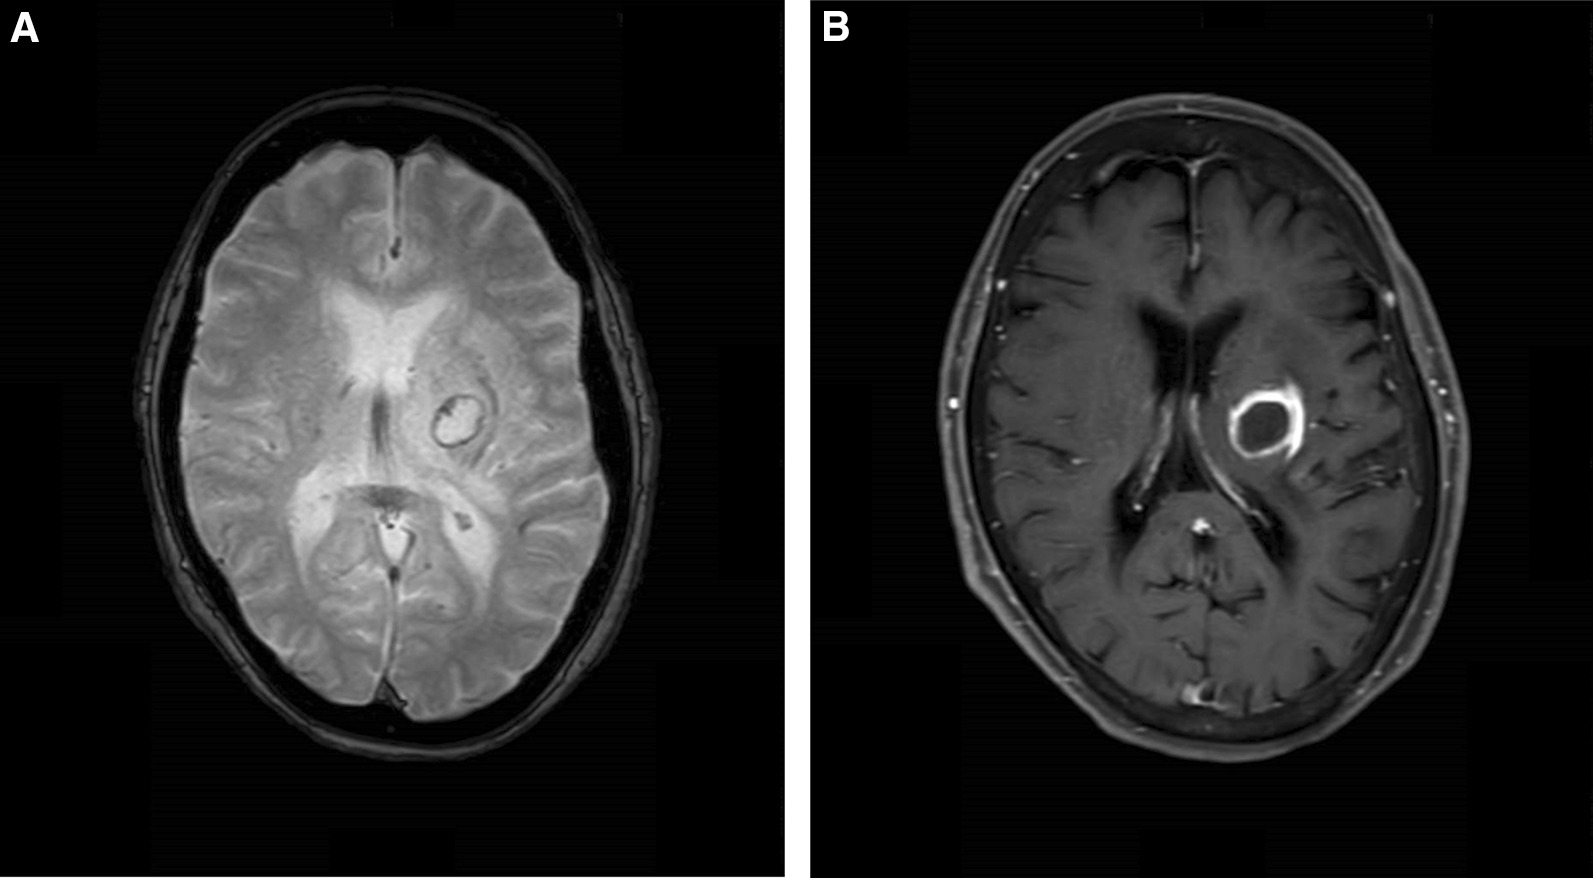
T2-weighted image shows rounded lesion with hypo-intense signal in the peripheral and hyper-intense signal in the center (left), and T1-weighted Gd-contrast enhancement shows well ring-enhanced cystic lesion (right)
